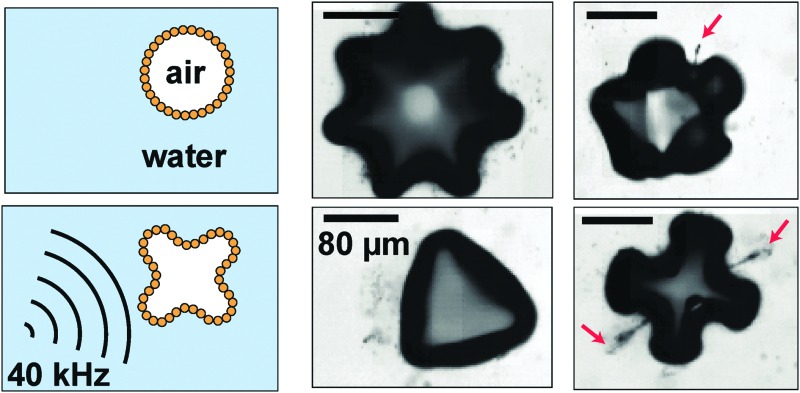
Ultrasound waves drive shape oscillations of particle-coated microbubbles. During the ultrafast, non-uniform deformation of the interface, particles are expelled from the antinodes of the shape oscillations.
